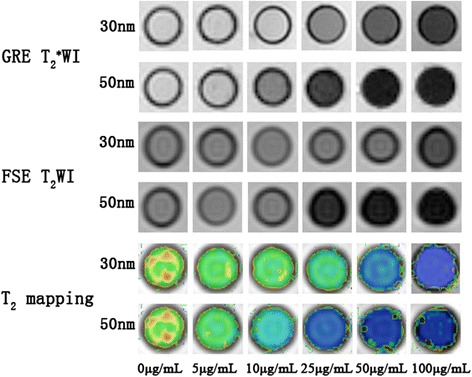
MR images of HUVECs labeled with different concentrations of 30 and 50 nm GMNPs. With increasing concentrations, the signal intensity of the HUVECs labeled with the 30 and 50 nm GMNPs decreased rapidly. Consistent with the trend of the signal intensity, the T_2_ relaxation time of the labeled cells decreased with increasing GMNP concentration.
